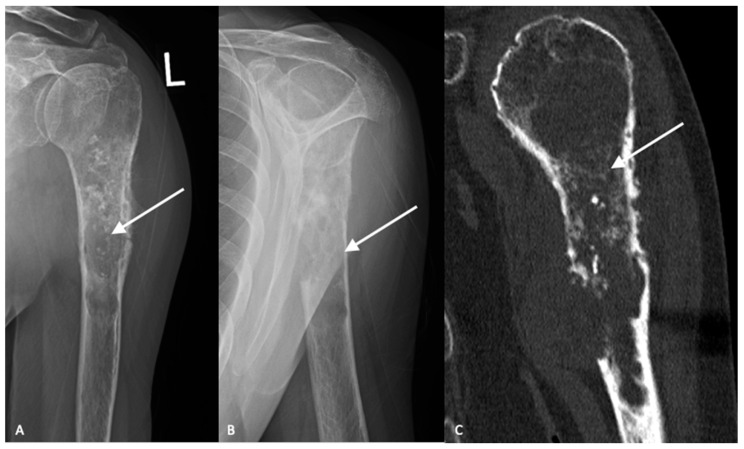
AP (A) and lateral (B) radiographs demonstrate an aggressive, heterogenous lytic lesion in the left proximal humerus with a wide zone of transition (white arrows). The lesion measures greater than 5 cm in length. Chondroid matrix is demonstrated. Periosteal reaction is present at the lateral aspect. There is a large area of cortical breakthrough at the anterior cortex, in addition to numerous areas of endosteal scalloping. Computed tomography (C) further demonstrates the cortical disruption and chondroid matrix formation (white arrow).
